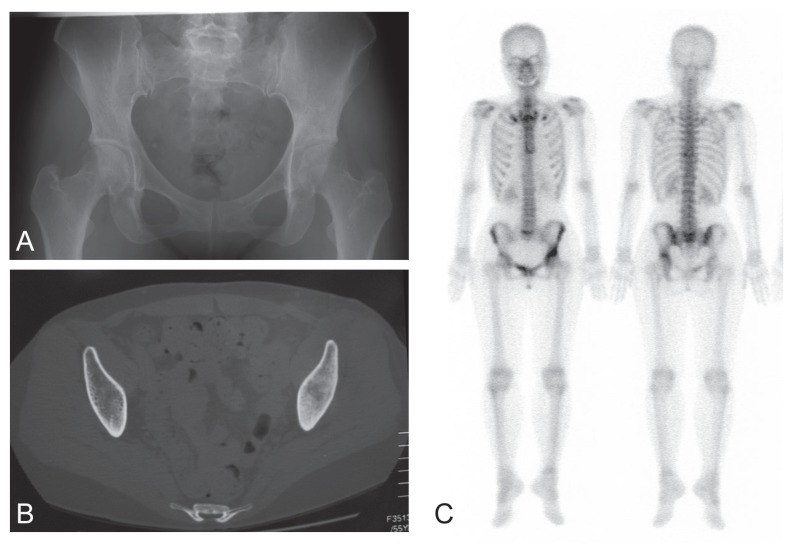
Plain radiographs show ill-defined sclerosis in the left para-acetabular region (A). CT shows diffuse irregular osteosclerosis in the left para-acetabular region, possibly associated with the fracture (B). Bone scintigraphy shows a markedly increased uptake in the left acetabulum (C).
